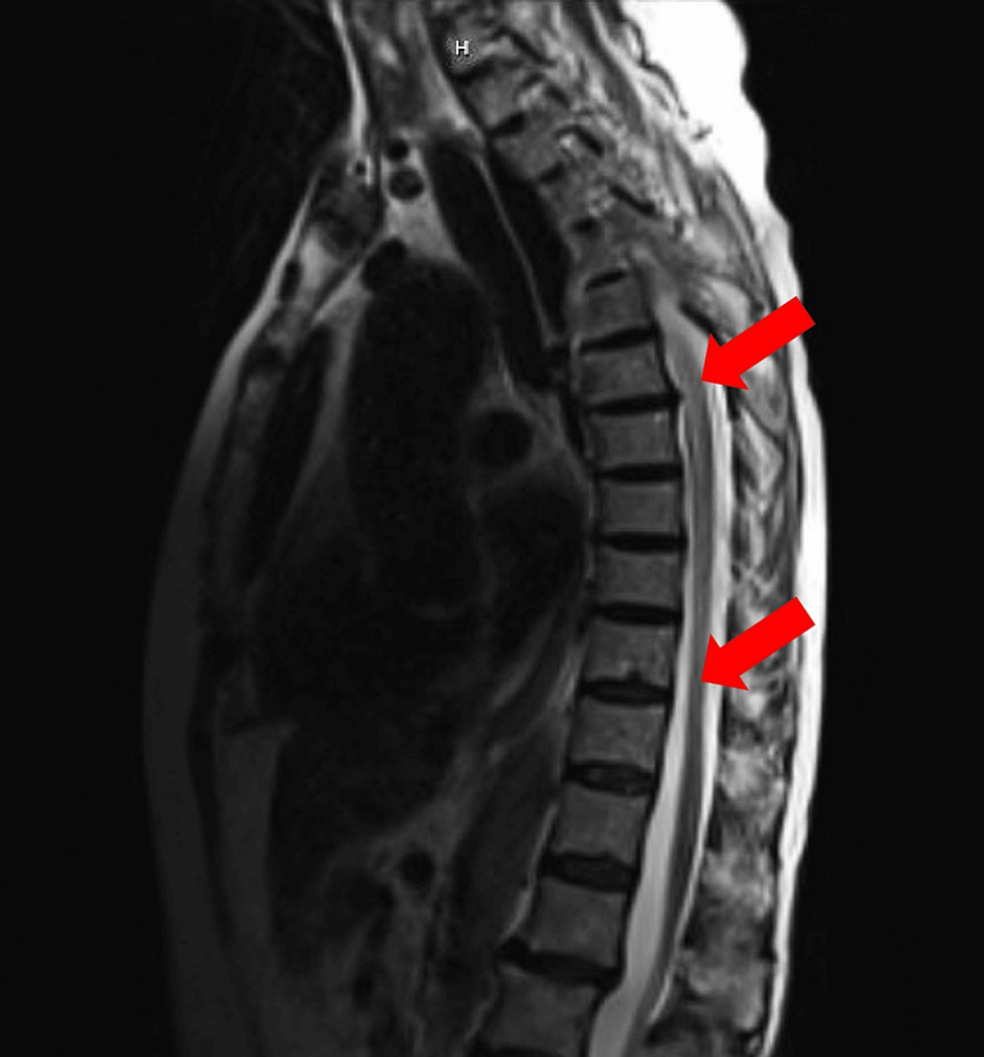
T2-weighted thoracic spine MRI, sagittal view, showing diffuse contrast enhancement (arrows) MRI: magnetic resonance imaging
